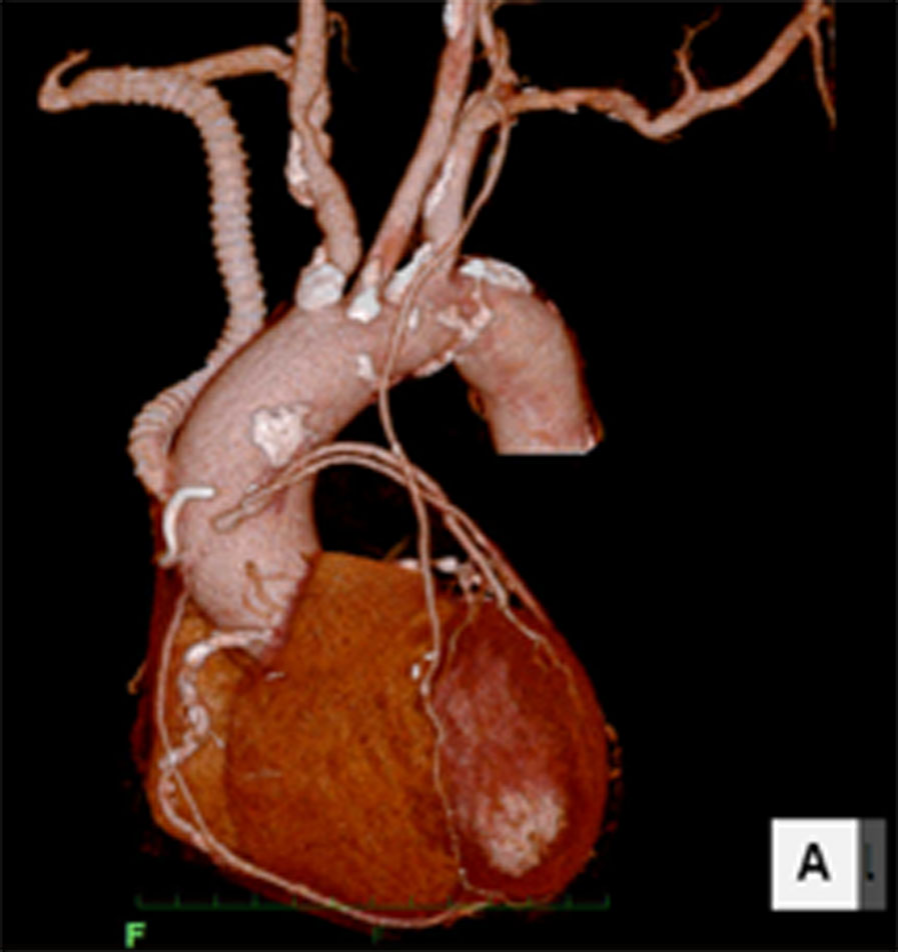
Postoperative computed tomography image showing patency of the aorto-right subclavian artery bypass and coronary artery bypass grafts
